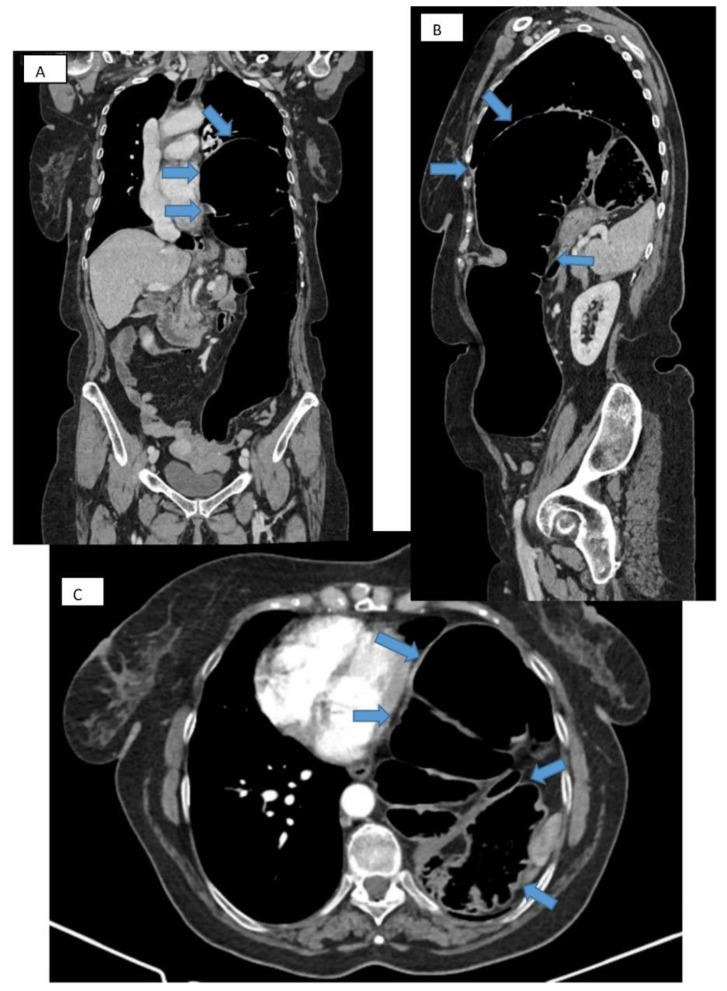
CT thorax with contrast. Frontal (A), sagittal (B), and transverse (C) section showing large diaphragmatic defect (Bochdalek hernia) with intrathoracic herniation of the colon.
